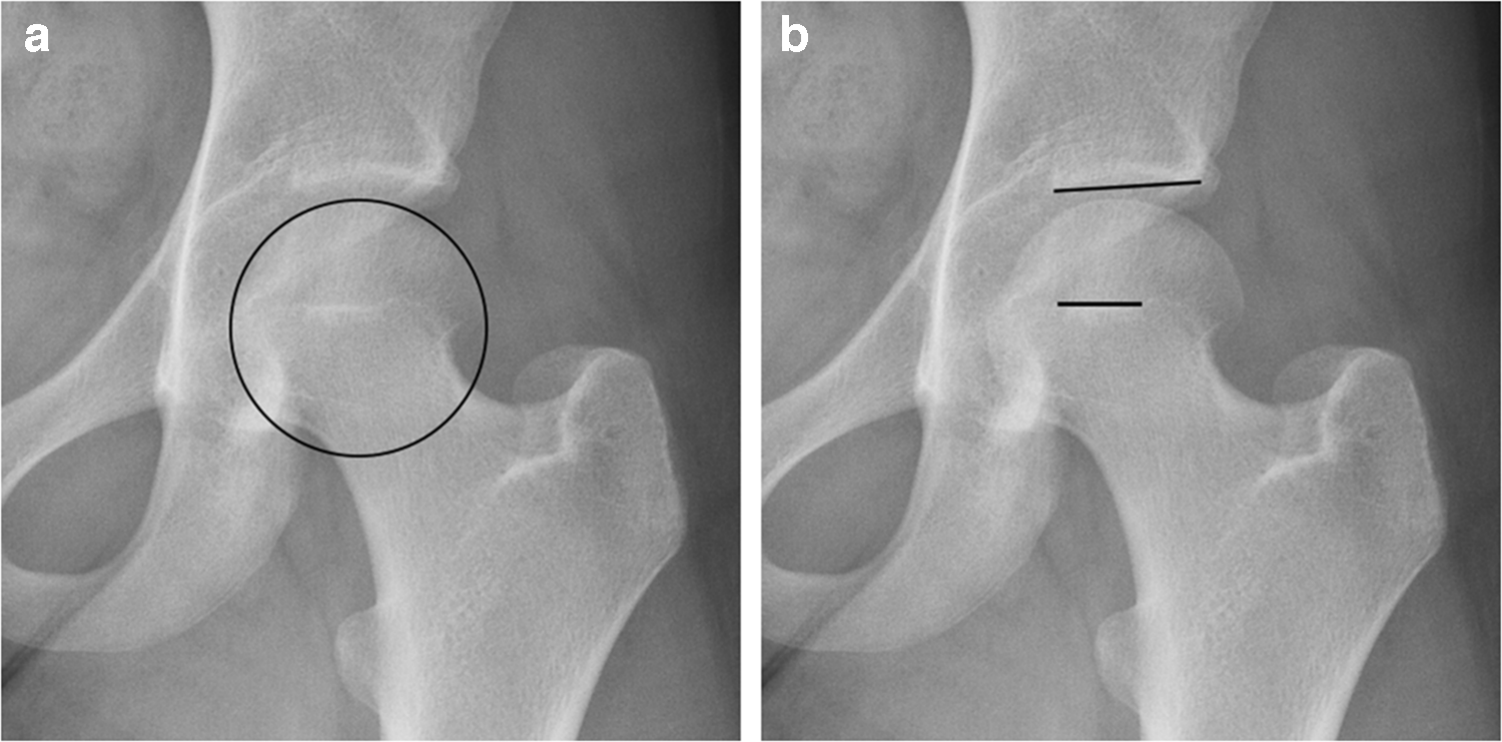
Radiograph in a 17-year-old female with clinical microinstability demonstrating a positive cliff sign (A) and a FEAR index of +5 degrees (B)
